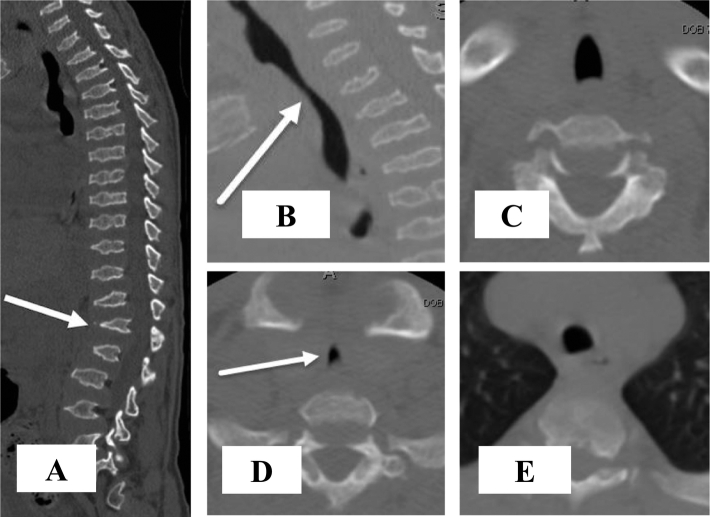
CT images of the spine, with attention to the vertebrae and trachea. A midline sagittal CT image of the spine (A) at 17 years of age shows typical features of MPS IVA leading to severe spinal stenosis, including: platyspondyly, endplate irregularity, anterior beaking of vertebral bodies, and mild retrolisthesis of L1 (arrow). T10-L4 spinal decompression and fusion was subsequently performed. Coned down sagittal image (B) from the same study with attention to the trachea shows narrowing of the trachea at the thoracic inlet (arrow), spanning approximately 3 cm craniocaudal. Coned down axial images just below the vocal cords (C), at the thoracic inlet (D), and 2 cm above the carina (E) show focal triangular shaped narrowing of the trachea (arrow) at the thoracic inlet. Tracheal cross-sectional area measures 109 mm^2^, 20 mm^2^, and 66 mm^2^ at these respective levels. There is mild tortuosity of the trachea in the coronal plane as well, not possible to show in a single image due to the oblique course and lack of 3D data set for multiplanar reconstruction. Narrowing of the thoracic inlet is due to bony changes of MPS IVA, including pectus carinatum and bulbous clavicular heads, as well as soft tissue crowding. Detailed vascular anatomy as a contributing factor cannot be delineated on this non-contrast CT of the spine.
